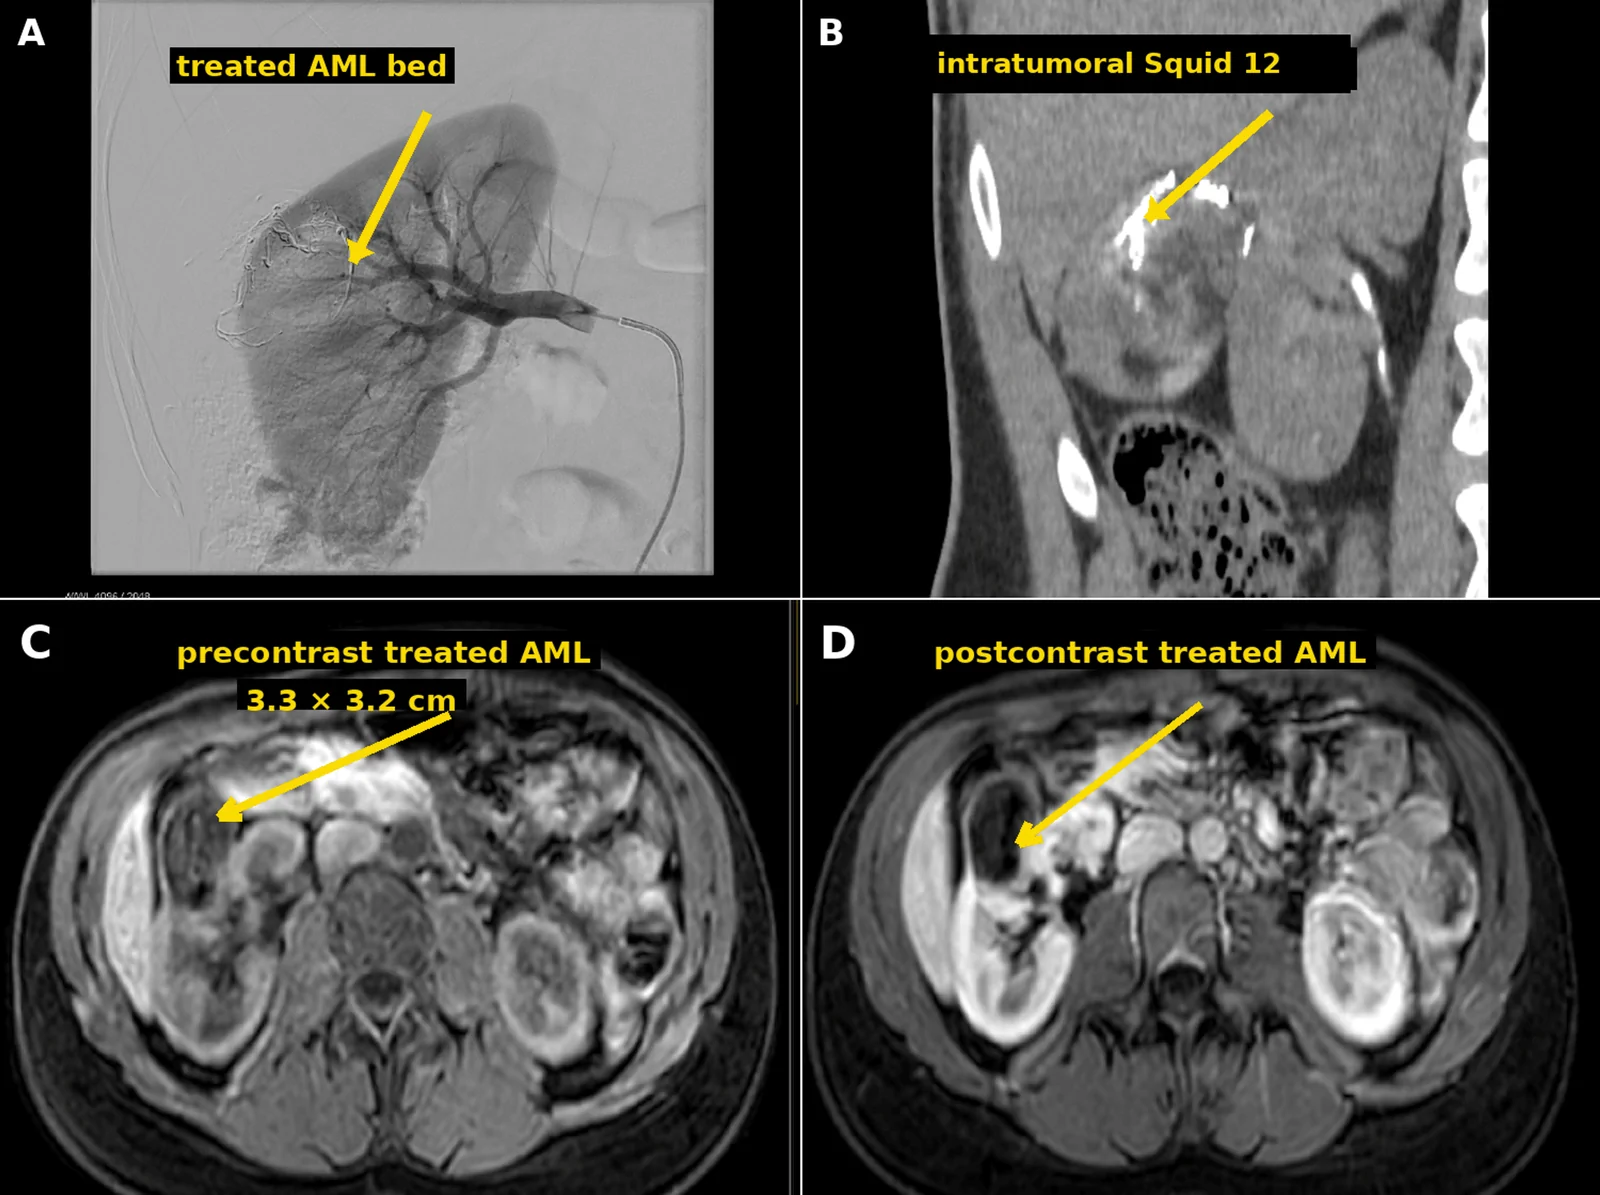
Procedural outcome and follow-up Completion DSA demonstrates the treated angiomyolipoma bed without residual tumor blush and with preservation of uninvolved renal parenchymal perfusion (A). Immediate noncontrast CT demonstrates radiopaque intratumoral Squid 12 without visible venous migration (B). At three-month follow-up, the displayed axial lesion dimensions are 3.3×3.2 cm on precontrast (C) and postcontrast (D) fat-suppressed T1-weighted MRI, with no residual enhancement. Yellow arrows identify the treated territory, embolic material, and residual treated lesion. DSA: digital subtraction angiography; CT: computed tomography; MRI: magnetic resonance imaging; AML: angiomyolipoma.
